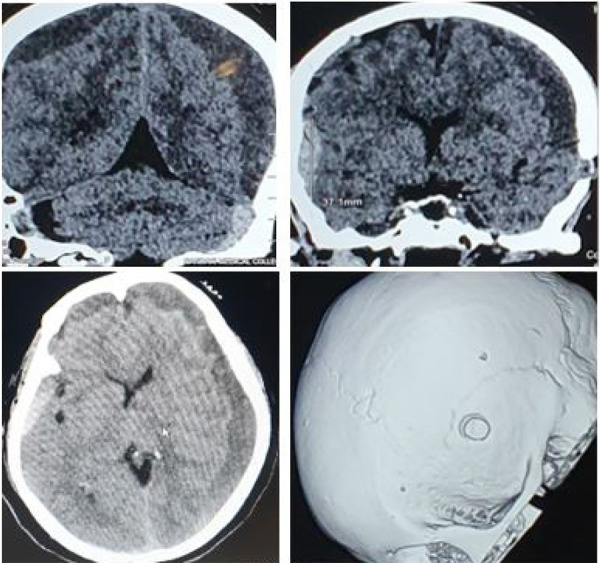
Initial non-contrast head CT showing bilateral CSDH with acute bleed on one side and a hole from previous trephination.
